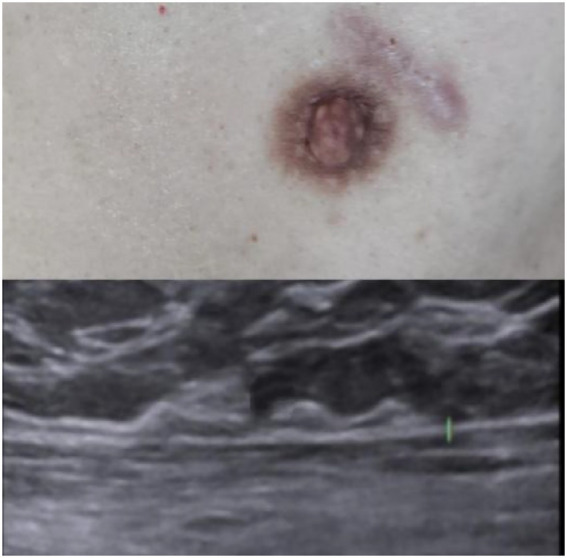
One-year postoperative follow-up of the left breast. Clinical examination revealed a well-healed scar with no infection or dehiscence, symmetric non-inverted nipples, and no palpable masses or lymphadenopathy. Color Doppler ultrasound (12-MHz linear probe) demonstrated homogeneous echotexture in bilateral mammary glands with normal vascularity, no residual lesions, and no focal hyperemia or abnormal color flow signals, consistent with BI-RADS 1 (negative findings).
